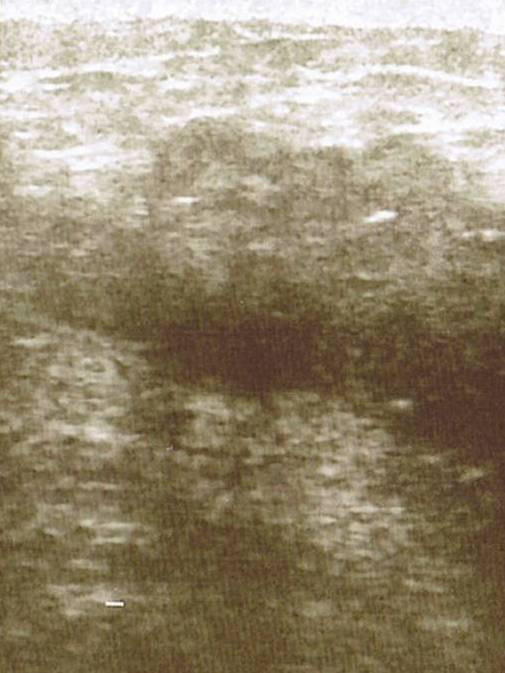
Ultrasound scan of the right breast showing showing the hypodense irregular areas in position close to the areola with some distal shadowing.
